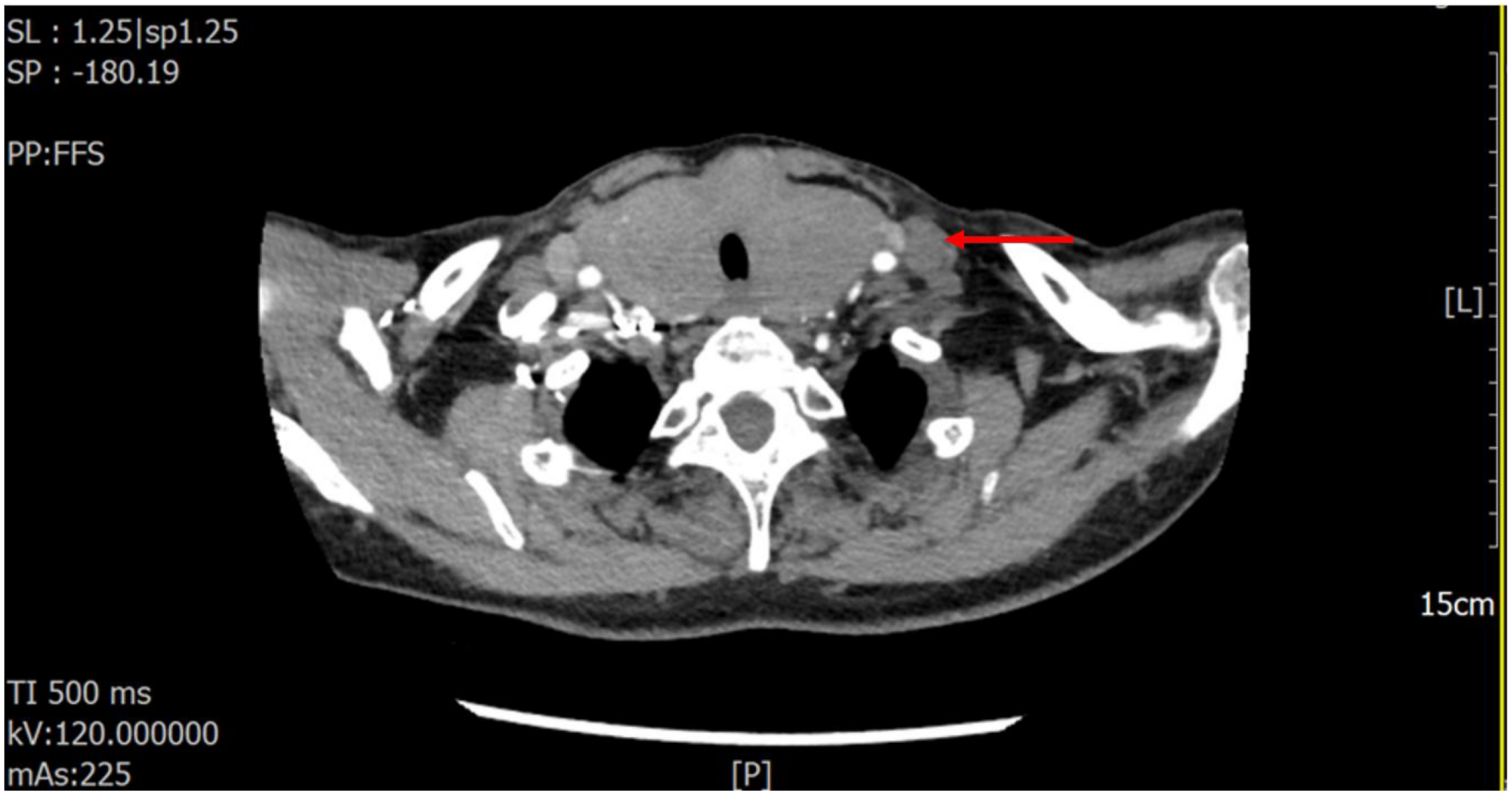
Contrast-enhanced CT of the neck revealing cervical lymphadenopathy (as indicated by the red arrow) and tracheal compression deformity.
